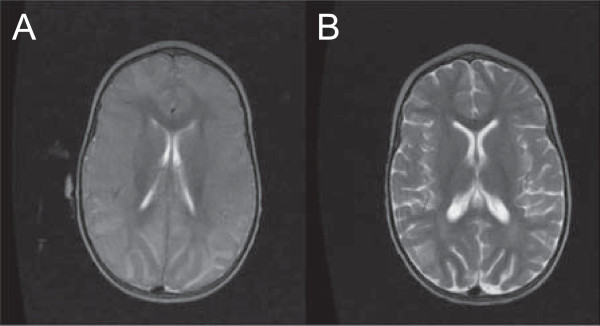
MRI of paediatric CM survivor from Malaŵi during the acute phase and at follow-up. Admission axial T2 scan (TR2960, TE122) of a 2 years old male Malaŵian child with retinopathy-positive CM performed at Queen Elizabeth Central Hospital in Blantyre, Malaŵi. There is severe brain swelling with total loss of sulcal markings and grey-white differentiation (A). The same child presents mild diffuse atrophy one month later during the post-discharge follow-up visit (B).
